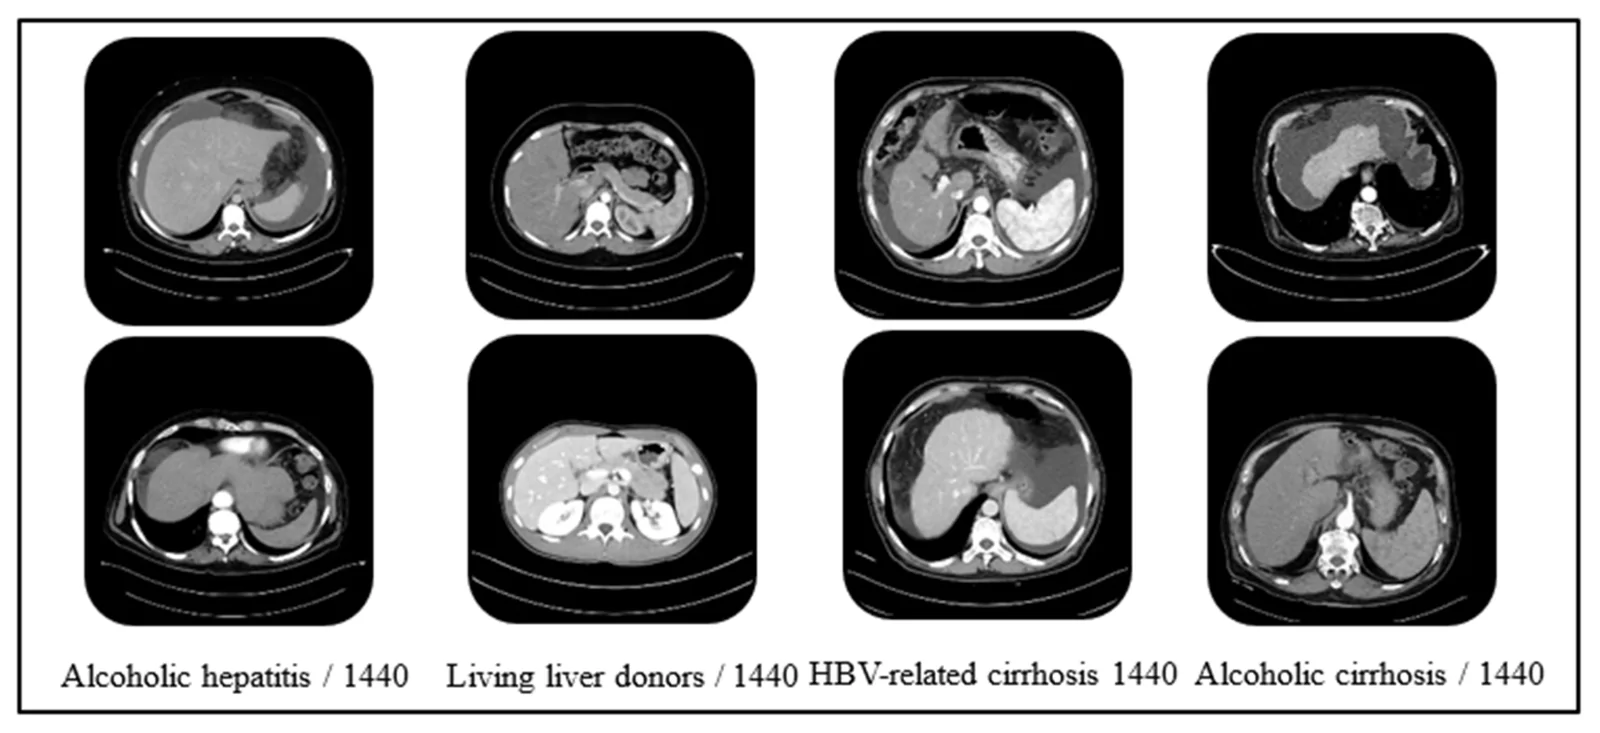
Representative CT images from the four study classes, with the number of images in each class.
